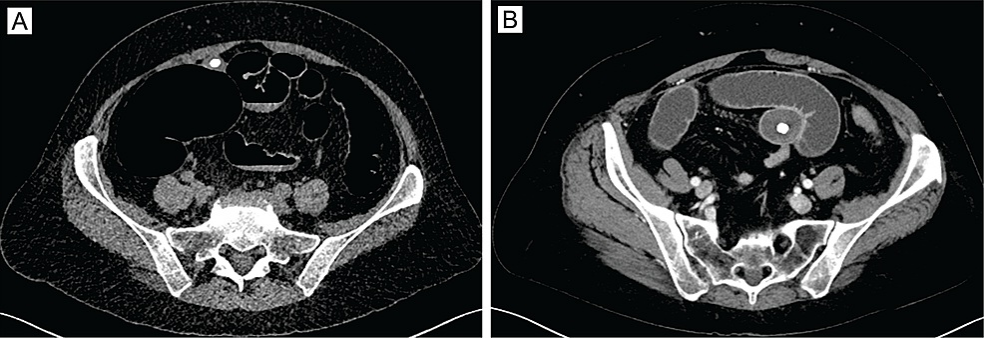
CT showing with Meckel's enterolith. (A) Historic imaging: radiopaque intraluminal mass was seen near the terminal ileum on a screening CT colonography performed six years prior to presentation. This mass was smaller than the mass that was removed. The lower-density periphery had grown in diameter, but the radiopaque core was identical in appearance. (B) Preoperative imaging: CT scan with IV contrast showing an obstructive mass with a calcified core in the terminal ileum. Small bowel is decompressed distal to the obstruction and dilated proximal to it.
